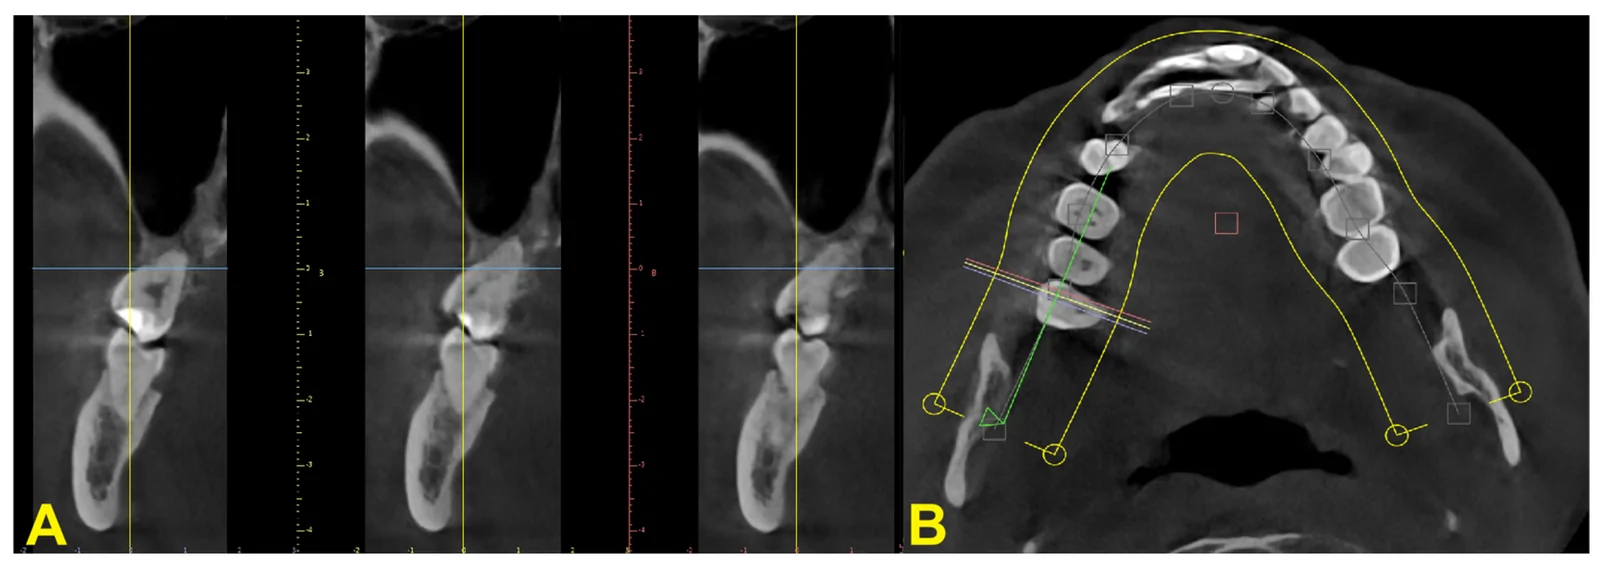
Cone-beam computed tomography of tooth 18 position: (A) cross-sectional views; (B) axial view.
